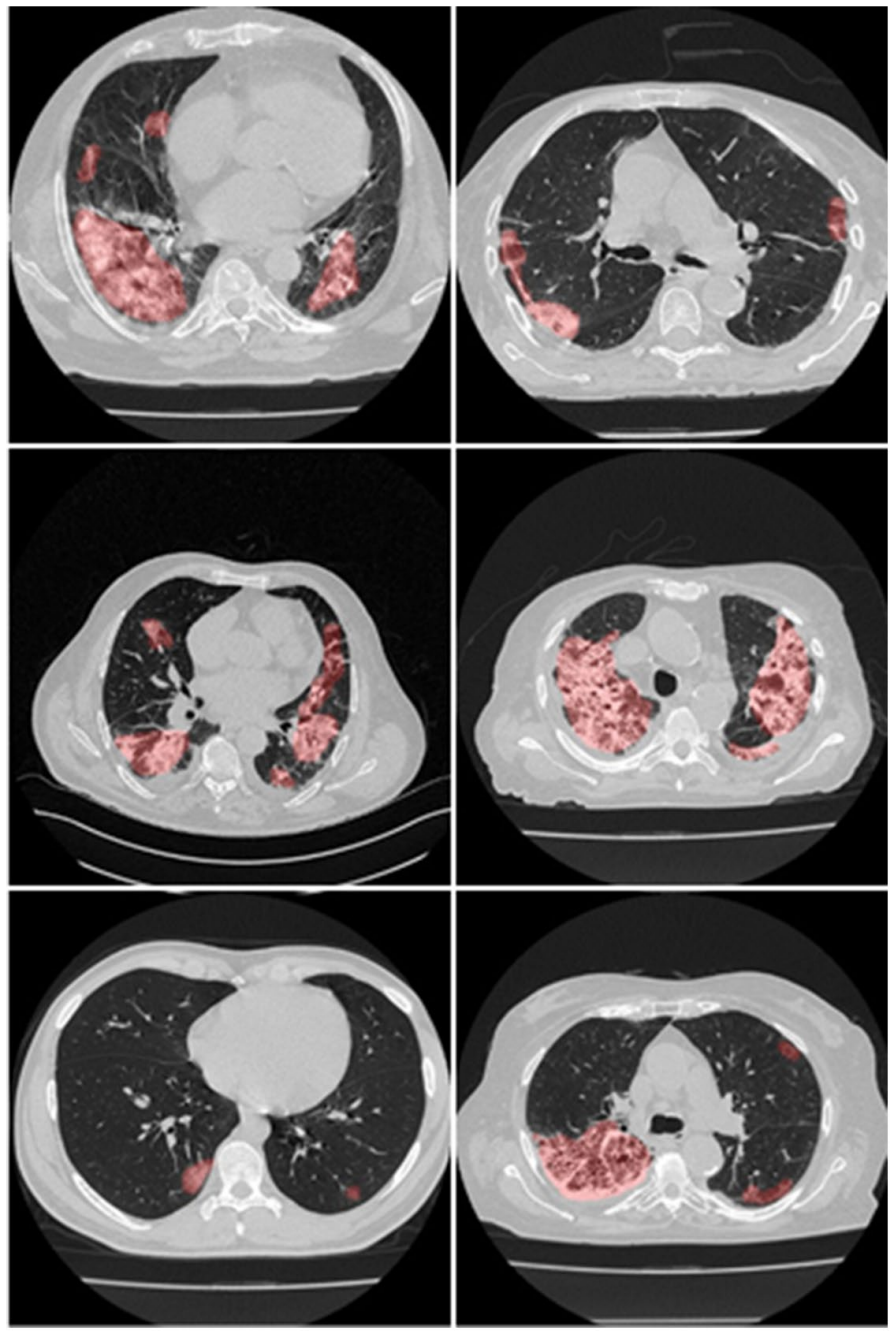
Manual overlays (red) on raw CT images.
